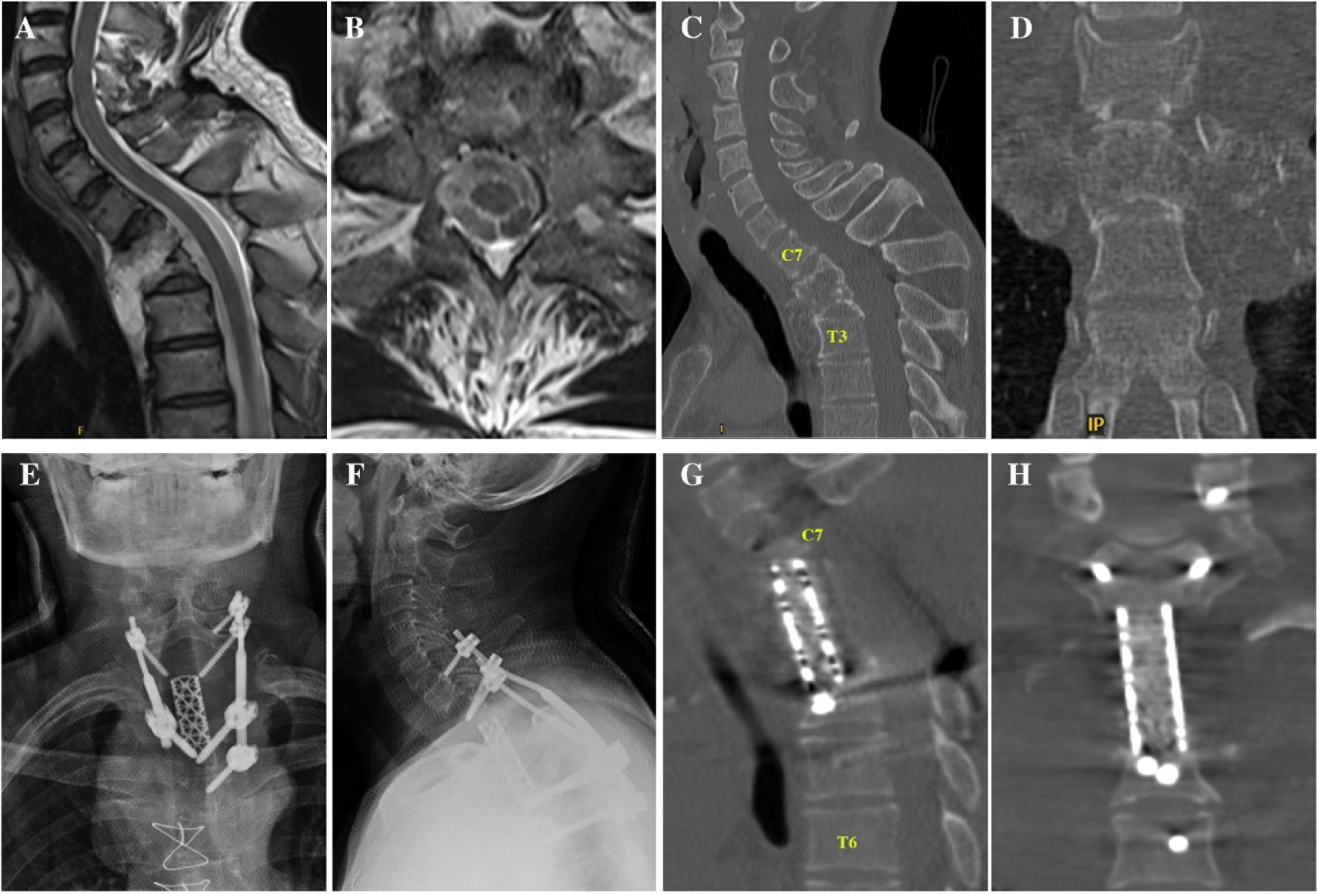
(A,B) Sagittal and axial MRI showing a tumour in the T1-2 kevel without obvious spinal cord compression. (C,D) Sagittal and axial CT showing tumour invasion of the T1–3 vertebral bodies. (E,F) Anteroposterior and lateral x-rays showing the region after total spondylectomy. (G,H) Sagittal and coronal CT image after total spondylectomy. CT, computed tomography; MRI, magnetic resonance imaging.
